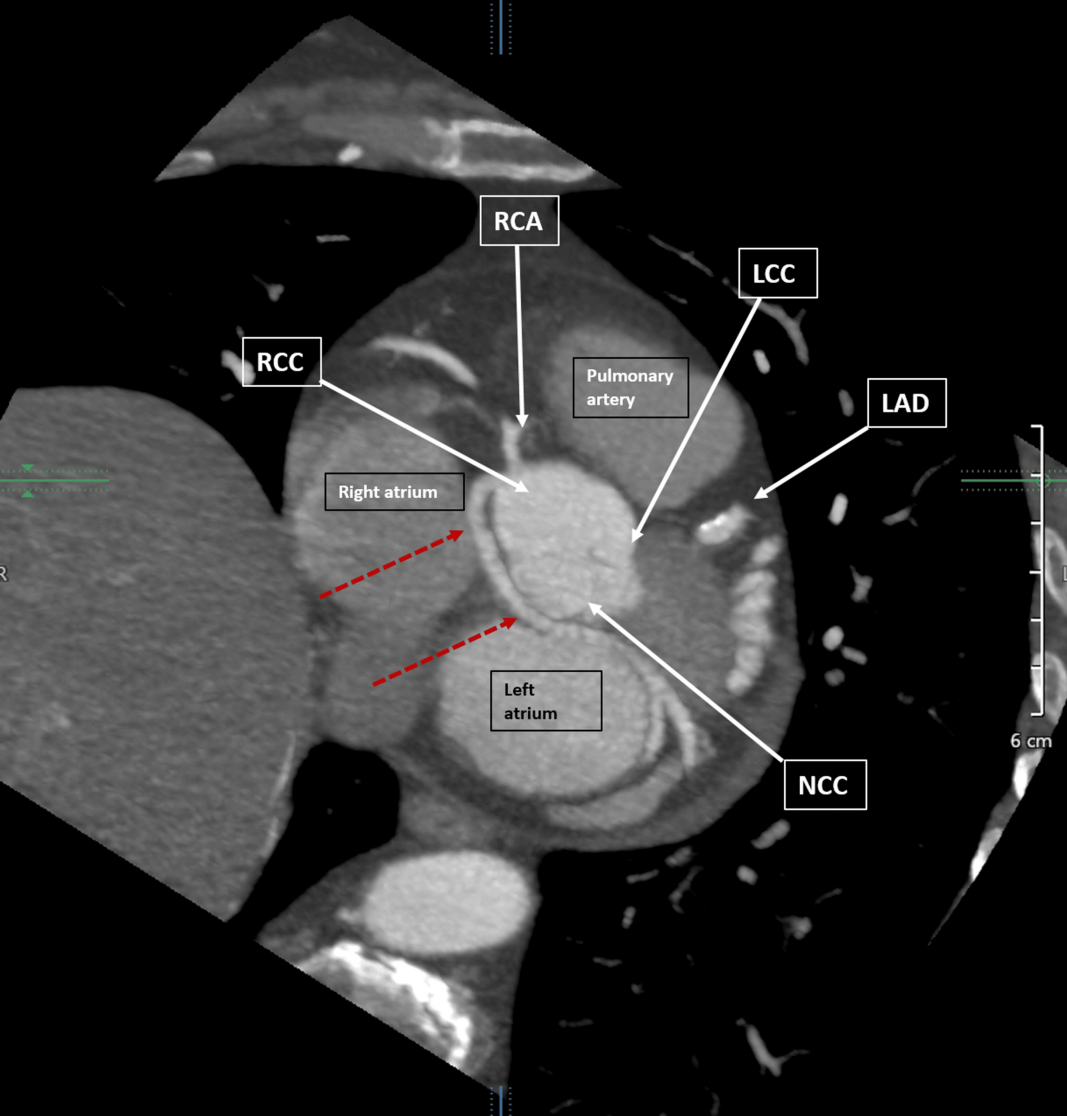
Coronary CT angiography (modified axial view) showing an anomalous left circumflex coronary artery (red dashed arrows) originating from the right coronary cusp and taking a retro-aortic course to the left atrioventricular groove. Coronary CT angiography (modified axial view) showing anomalous left circumflex coronary artery (LCx) originating from the right coronary cusp (RCC) and taking a retro-aortic course to the left atrioventricular groove (red dashed arrows). The ostium of the LCx is separate from the ostium of the right coronary artery (RCA). The left anterior descending coronary artery (LAD) is seen in the anterior interventricular groove with <25% stenosis due to calcified plaque. Also noted in the figure are the left coronary cusp (LCC) and the noncoronary cusp (NCC).
